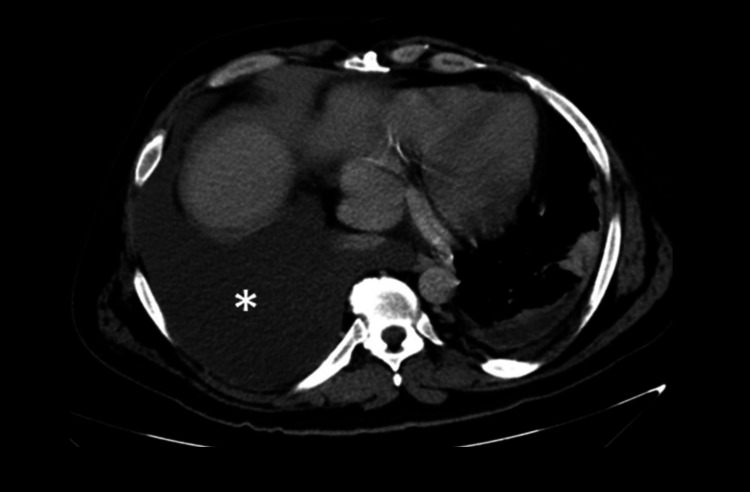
Contrast-enhanced chest computed tomography Axial contrast-enhanced CT of the chest demonstrating a large right-sided pleural collection with high attenuation (asterisk), compatible with hemothorax, occupying a substantial portion of the right hemithorax and causing compressive atelectasis of the adjacent lung parenchyma.
